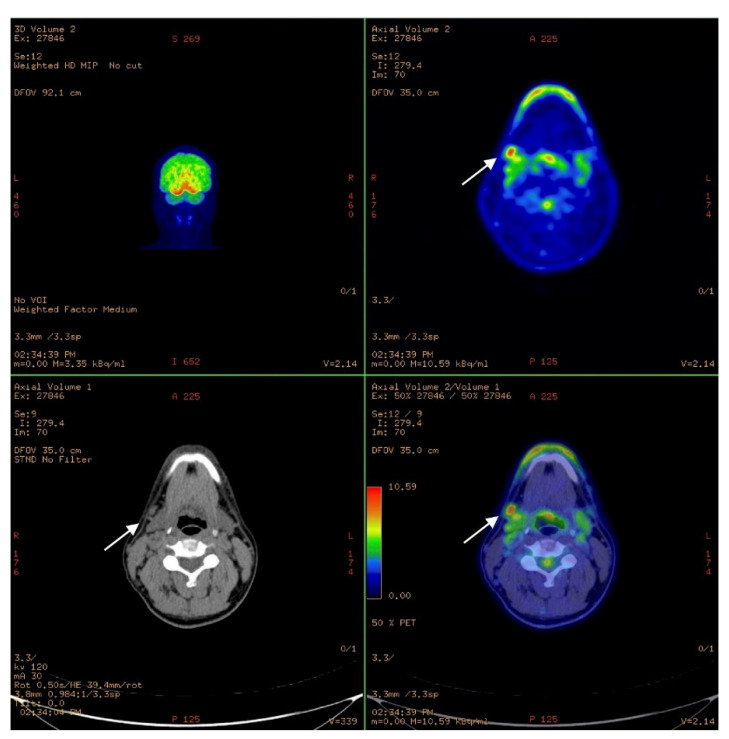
PET/CT (Positron emission tomography/Computed tomography) scan shows positive cervical lymph nodes at level IIa (white arrows).
